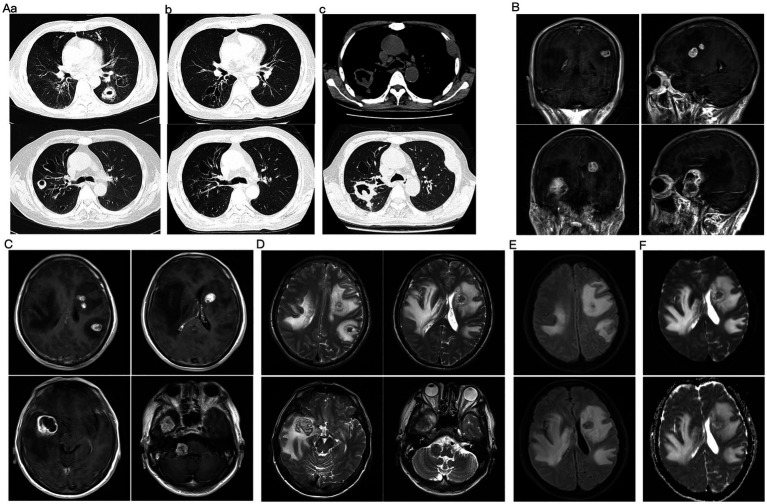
Longitudinal chest CT evolution and brain MRI on current admission. (A) Chest CT evolution. (a) Initial CT at IPA diagnosis showing thick-walled cavities in the dorsal segment of the left lower lobe and the anterior segment of the right upper lobe (measuring approximately 35 × 28 mm and 25 × 20 mm), with surrounding infiltrates. (b) Follow-up CT after 4 months of antifungal therapy demonstrating complete resolution of cavities with residual bronchiectasis and fibrotic changes. (c) CT on current admission revealing a new, irregular cavitary mass (approximately 65 × 29 mm) crossing the oblique fissure between the upper and lower lobes of the right lung, distinct from the prior sites of infection. (B–F) Brain MRI on current admission. (B,C) Post-contrast T1-weighted images showing multiple enhancing nodular lesions in both cerebral hemispheres and the right cerebellum, with significant mass effect and leftward midline shift. The largest lesion, located in the right temporal lobe, measures approximately 27 × 33 mm and demonstrates a lobulated contour. (D) T2-weighted image demonstrating the lesions as hypointense with extensive surrounding vasogenic edema. (E) FLAIR image highlighting the perilesional edema and mass effect. (F) DWI and corresponding ADC map. ADC map showing relatively low signal intensity at the lesion rim, suggesting hypercellularity, while the larger lesion demonstrated central hyperintensity on DWI, consistent with central necrosis.
